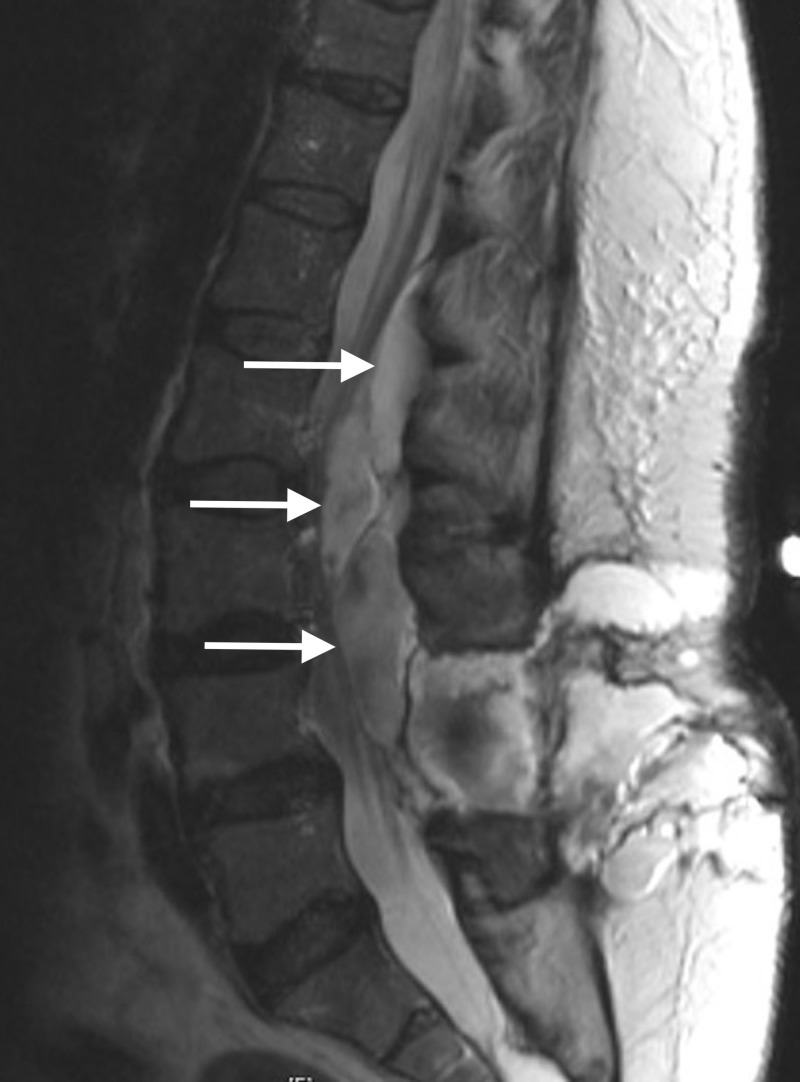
Sagittal T2 TSE MRI of the Spine TSE: turbo spin echo; MRI: magnetic resonance imaging
